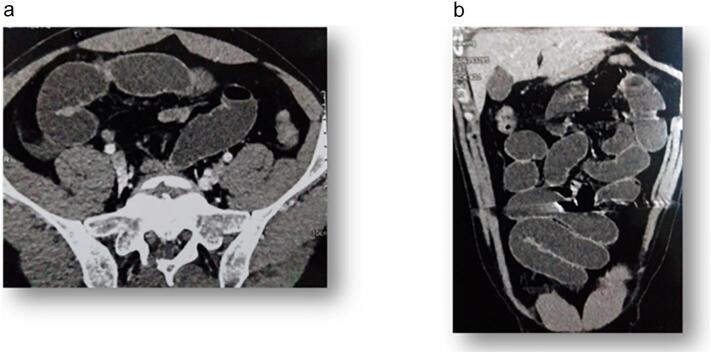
a: CT scan: diffuse dilatation of the small bowel loops with a collapsed colon lumen. b: CT scan: the transition zone appeared to be at the distal ileum regarding the paravertebral space of L5-S1.
